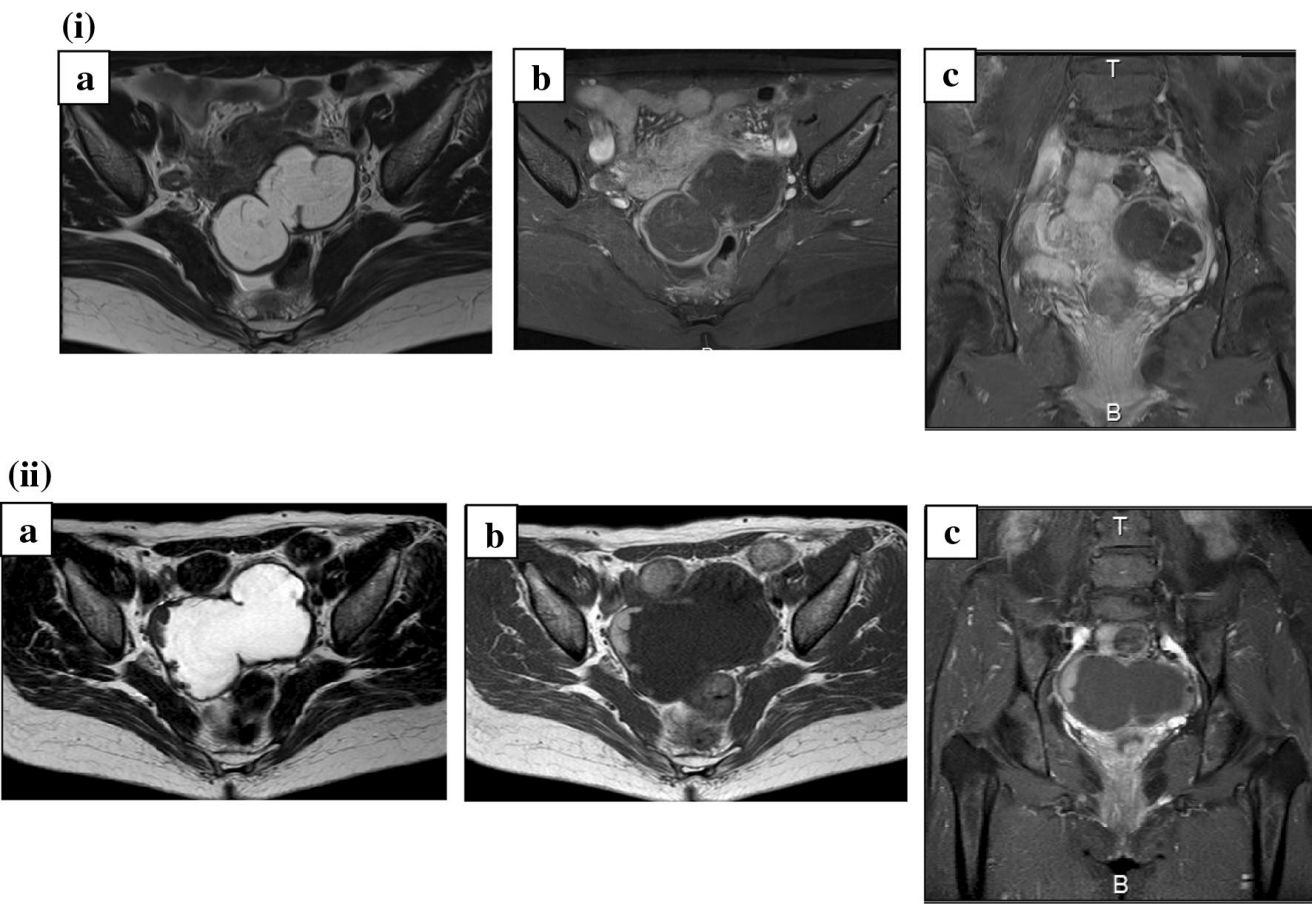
MRI findings. i At the first time. MRI showed a bilocular 8 cm mass with thick wall posterior to the uterus and demonstrated bleeding or mucinous component. *a* T2WI, *b* T1WI fat suppression, *c* Gd enhancement. ii 6 years later. MRI showed a 9×6 cm pelvic mass, including partial papillary nodules with contrast enhancement and little ascites. a T2WI, b T1WI, c Gd enhancement
